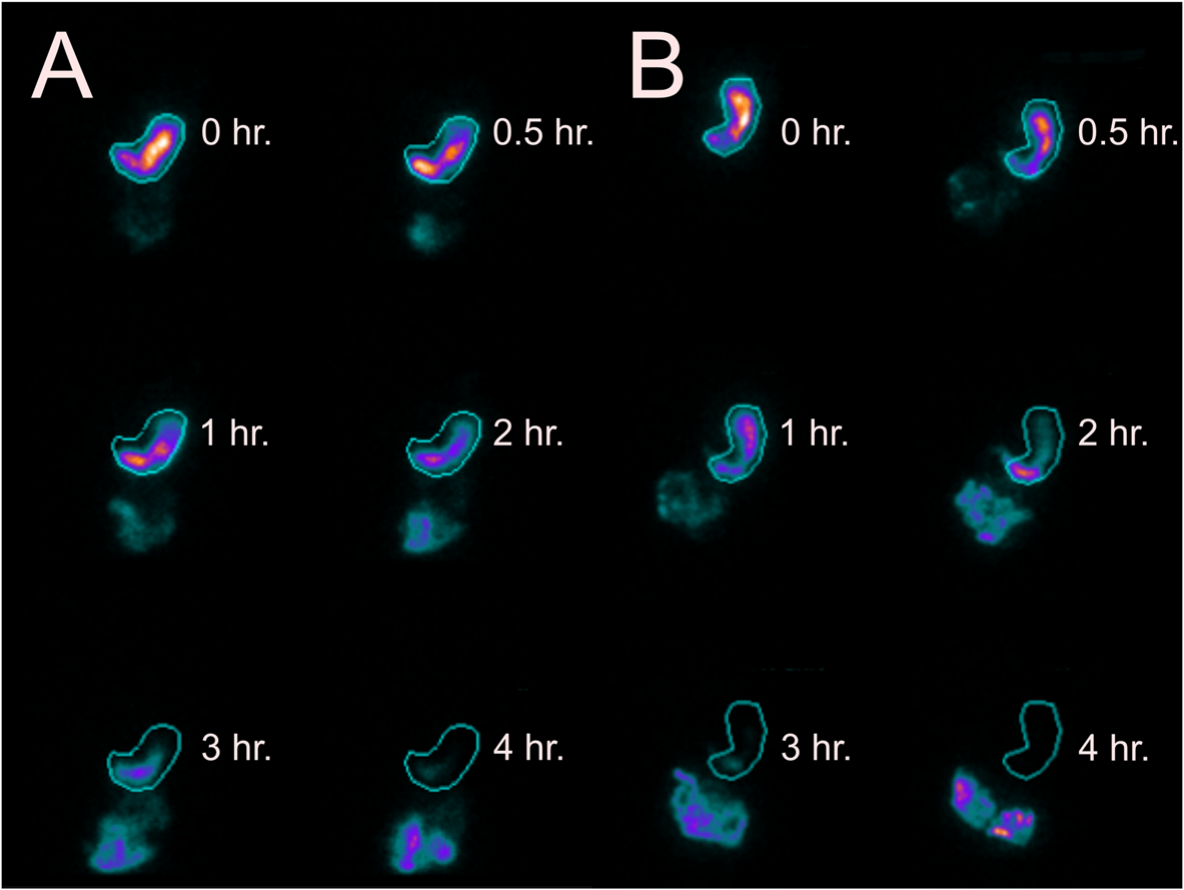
Paired anterior images of the 4-h gastric emptying scintigraphy protocol acquired from a healthy female participant. (a) ^99m^Tc-SC, and (b) ^99m^Tc-SP
